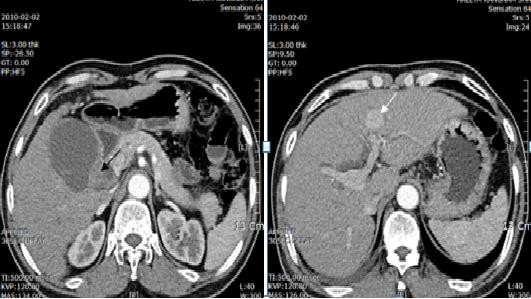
CT imaging results. a) A distended gallbladder with wall thickening and tiny gallstones in the gallbladder (black arrow) is evident. b) A tumorous lesion heterogeneously enhanced by contrast media in the left lobe of the liver (white arrow) is evident.
